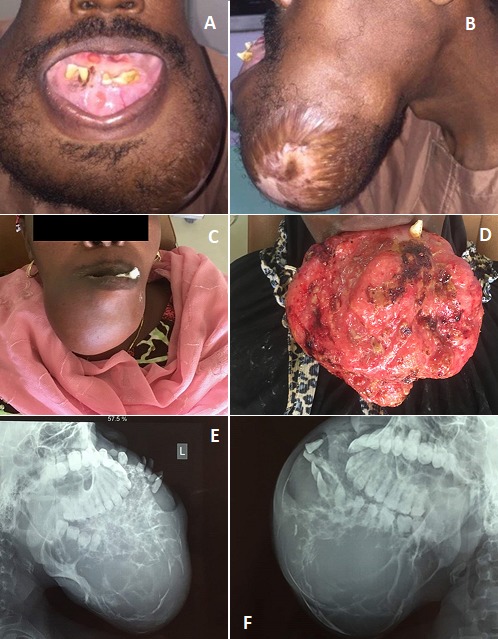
Clinical and radiological features of ameloblastic carcinoma. Ameloblastic carcinoma may presents as an aggressive expansile swelling of the jaws (A-C), and can present an exophytic extraoral mass (D). Radiographically, it can present as a jaw mass will an ill-defined border (E, F)
